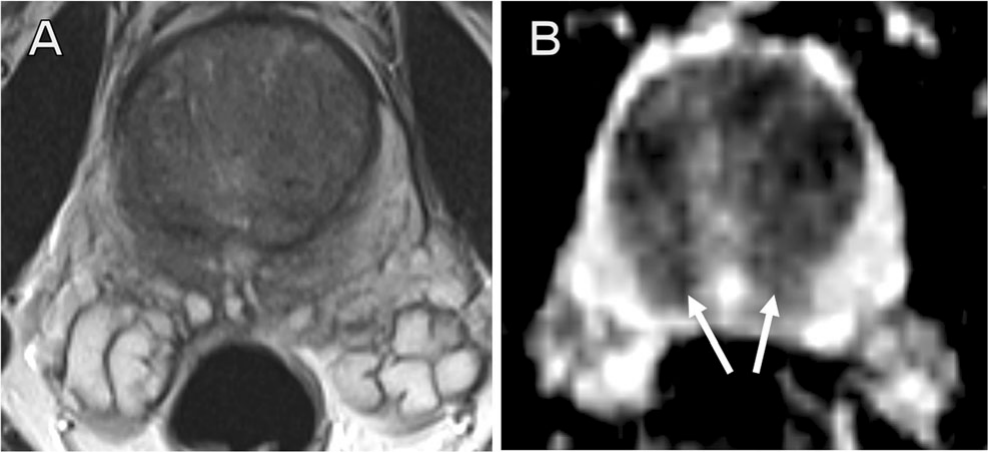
False-positive external report. A 50-year-old patient with16.6 ng/ml PSA and previous negative TRUS biopsy. External report described high probability targets bilaterally and medially at the base peripheral zone. Second read called a negative MRI, with normal central zone demonstrating low T2 signal (a) and low signal on ADC maps (b, *arrows*). Subsequent transperineal template biopsy showed all 24 cores to be benign
